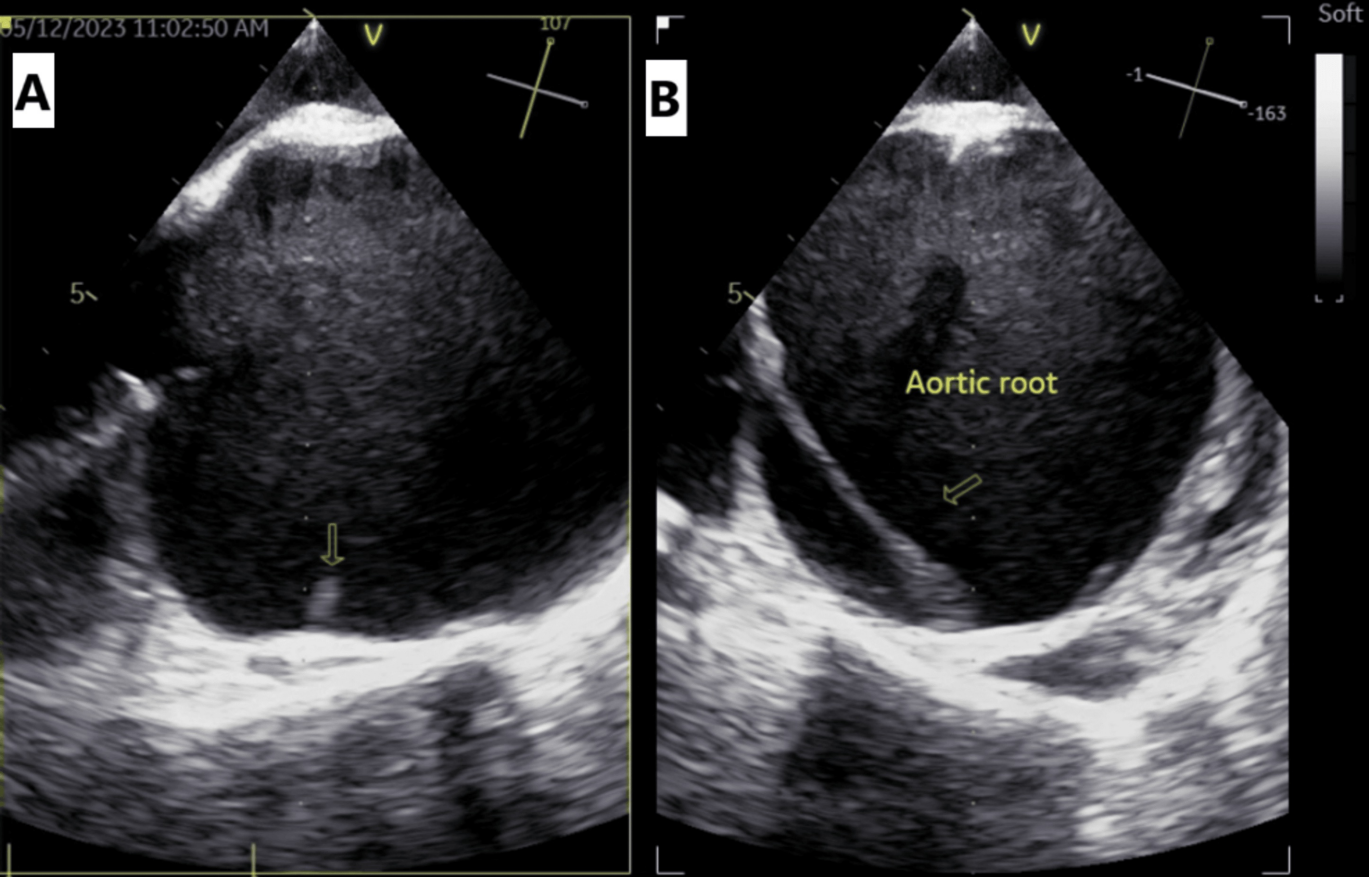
(A,B) TEE showing a dilated aortic root (7.3 cm) with a small intimal flap localized at the aortic root. TEE: transesophageal echocardiography
